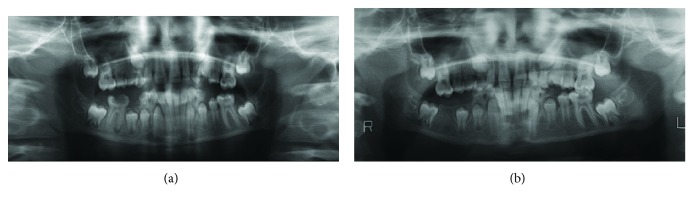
Orthopantomographic image showing a deep caries cavity in the right mandibular first molar tooth, a radiolucent area in its mesial root, and subperiosteal new bone formation below the lower border of the mandible (a). Orthopantomographic image taken four months after tooth extraction showing the return of normal bone contours (b).
